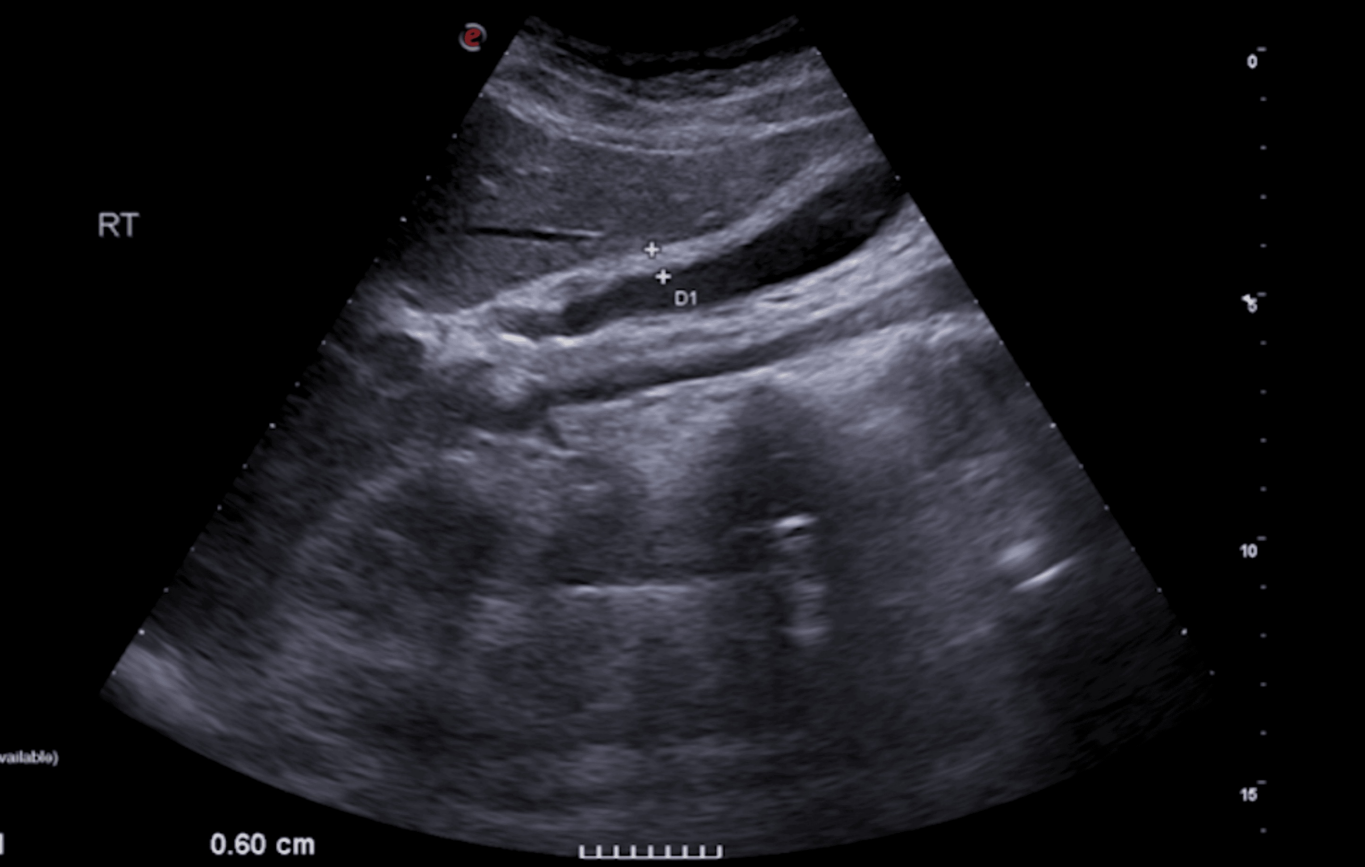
Ultrasonographic image of the gallbladder on the third day of hospitalization, showing a thickened wall (6 mm) without evidence of gallstones, suggestive of acute acalculous cholecystitis (ACC)
